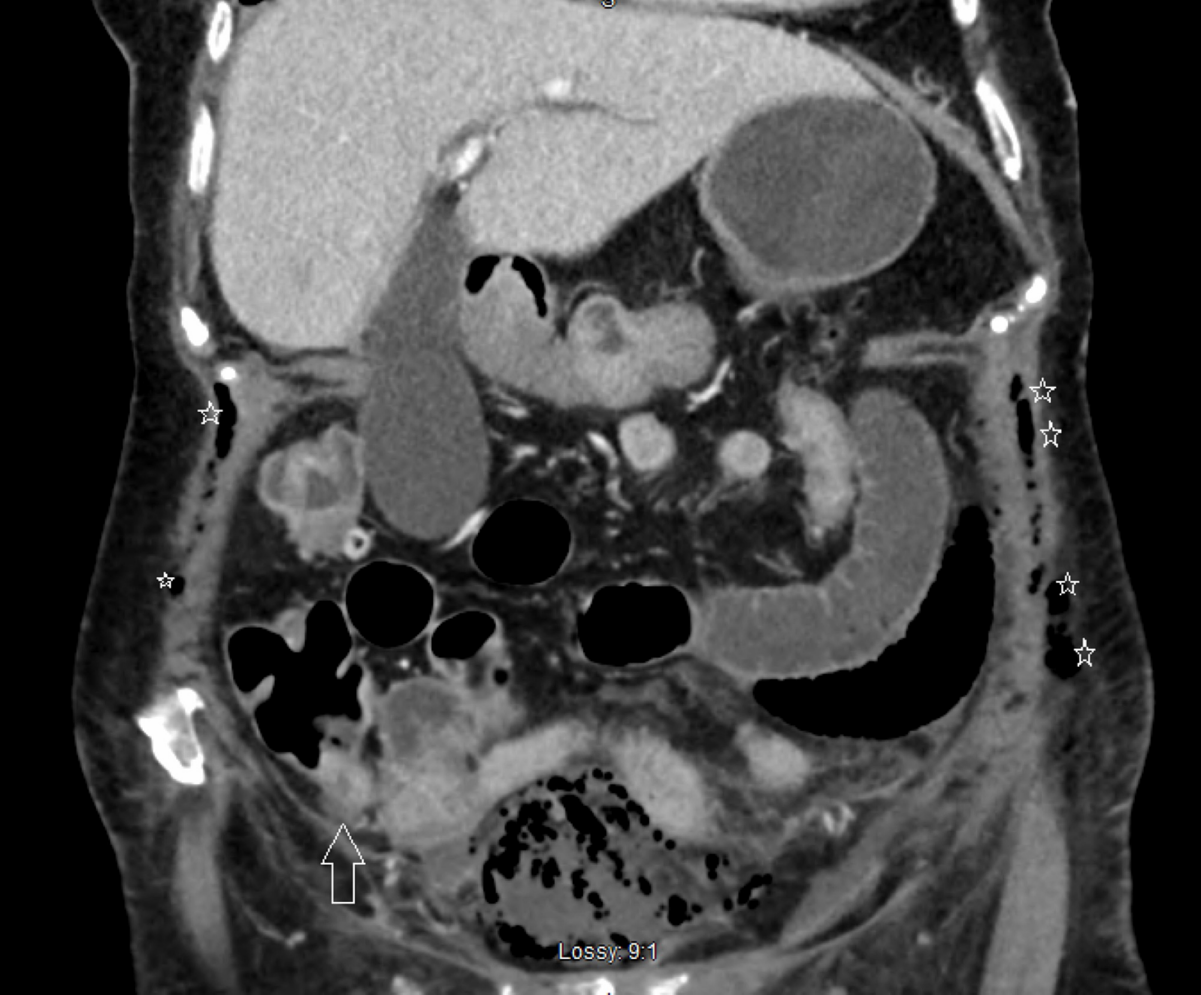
CT scan – coronal view – a thickened appendix (white arrow) with subcutaneous emphysema seen on both sides of the abdominal wall (white stars)
